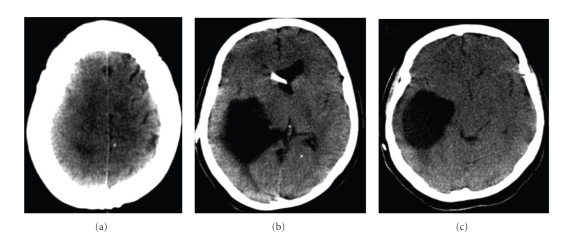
Noncontrast CT scans (2006). Ventriculo-peritoneal shunt with hypertensive obstructive hydrocephalus.
